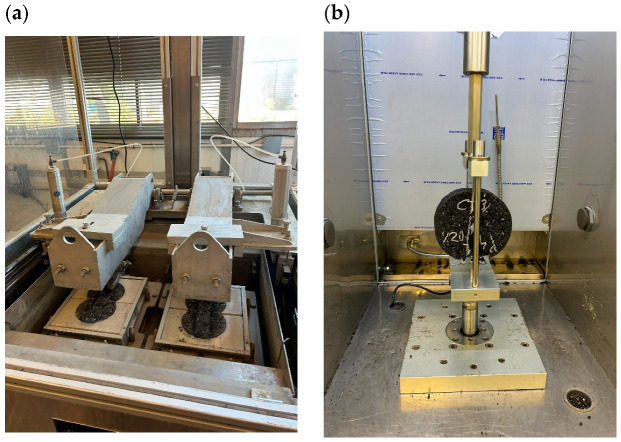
(a) Hamburg wheel track test setup; (b) IDEAL-CT Test Setup.
